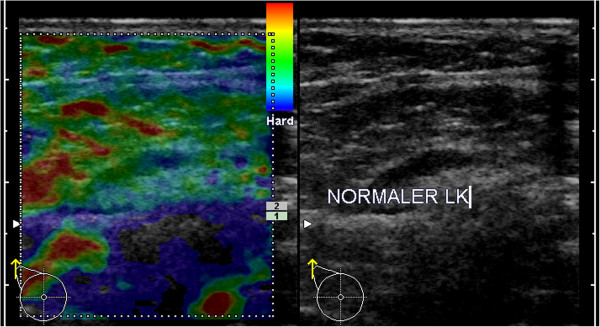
Example for B-mode ultrasound and elastogram of a healthy, reactive LN. In B-mode ultrasound, the cortex of the LN is slightly enlarged (maximum ~3.5mm). The predominant color of the medulla is turquoise (to green) and the cortex is mainly green. Applying the criterion of a blue cortex, this case would be a true-negative.
